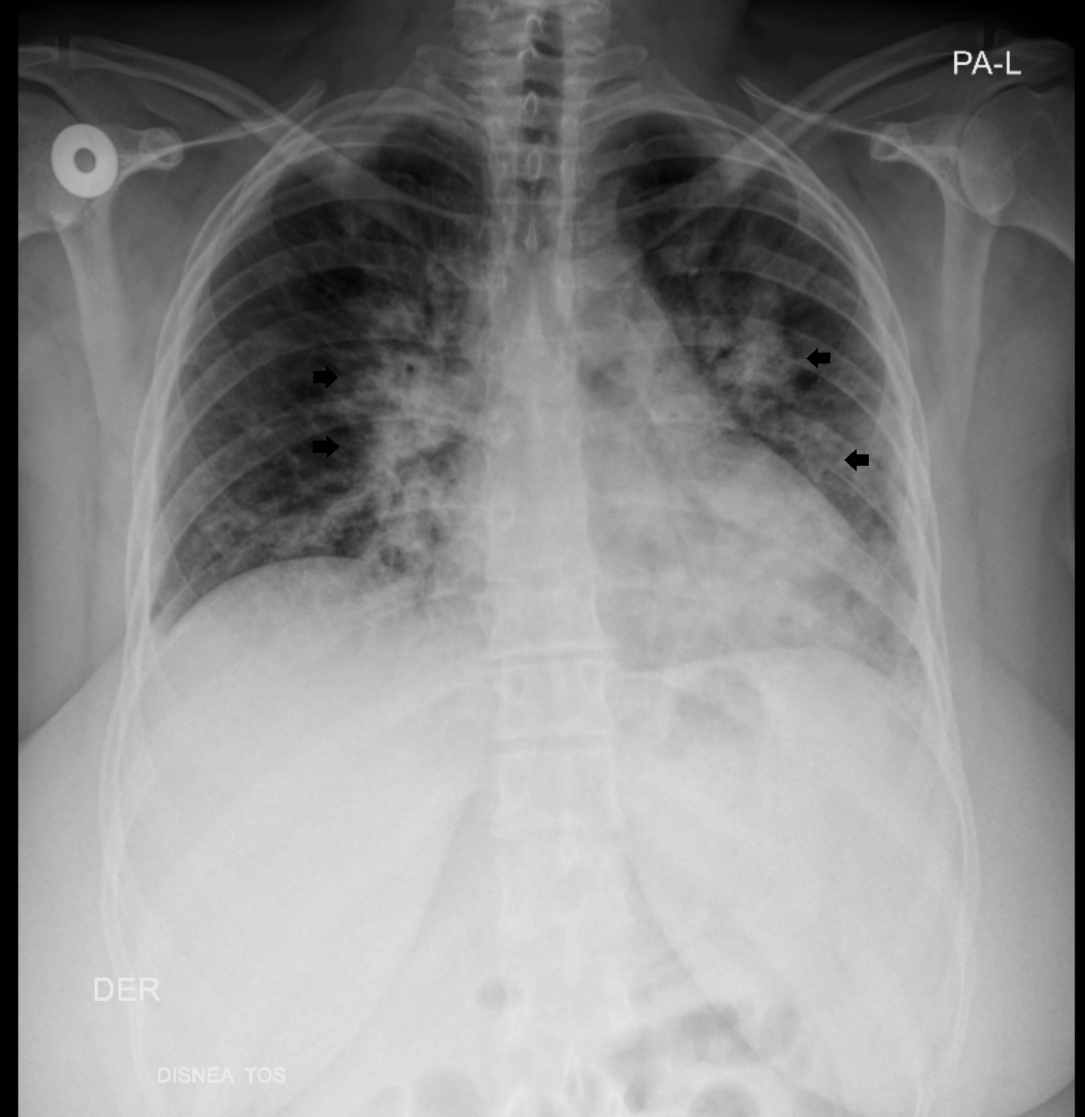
Chest X-ray: with diffuse alveolo-interstitial infiltrate predominantly on the left. Black arrows: increased pulmonary radiolucency due to diffuse alveolar-interstitial infiltrate.
